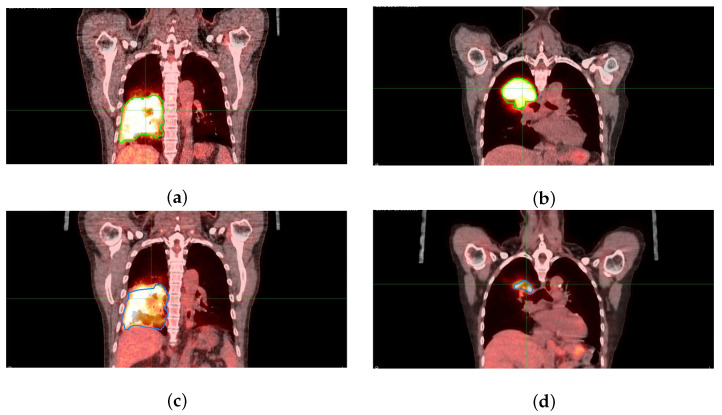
FDG-PET/CT images for an example PET non-responder patient (a,c) and PET responder patient (b,d), acquired pretreatment (a,b) and mid-treatment (c,d). Tumor volumes are displayed as blue/green contours.
